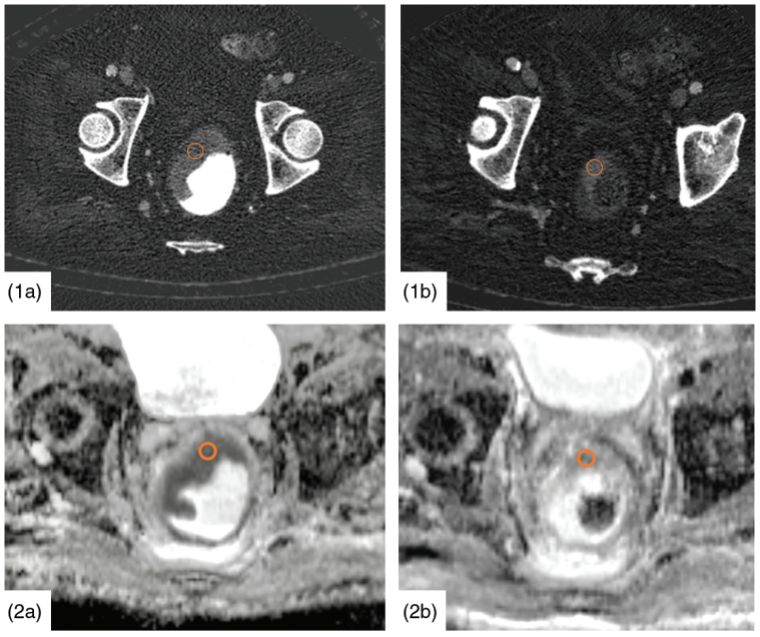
Dual-layer CT iodine imaging (1) of the tumor and MRI (2) before (1a, 2a) and after (1b, 2b) RCT. IC and ADC are measured via corresponding ROIs in CT and MRI images. The tumor stadium was rated uT3 (before RCT) and yT2 (after RCT), respectively. ADC, apparent diffusion coefficient; CT, computed tomography; IC, iodine concentration; MRI, magnetic resonance imaging; RCT, radiochemotherapy; ROI, region of interest.
